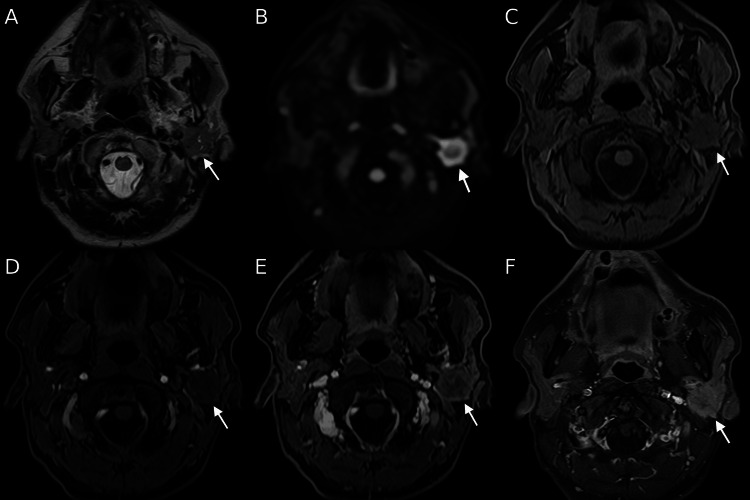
Magnetic resonance imaging (MRI) of the left parotid lesion The lesion (arrow) appeared slightly hyperintense on T2-weighted imaging (A) and hyperintense on diffusion-weighted imaging, with an apparent diffusion coefficient of approximately 0.9 × 10⁻³ mm²/s (B). On dynamic contrast-enhanced sequences, the lesion (arrow) demonstrated mild, progressive enhancement in the early phase and relatively marked enhancement in the delayed phase (C-F). Percutaneous biopsy confirmed inflammatory myofibroblastic tumor.
